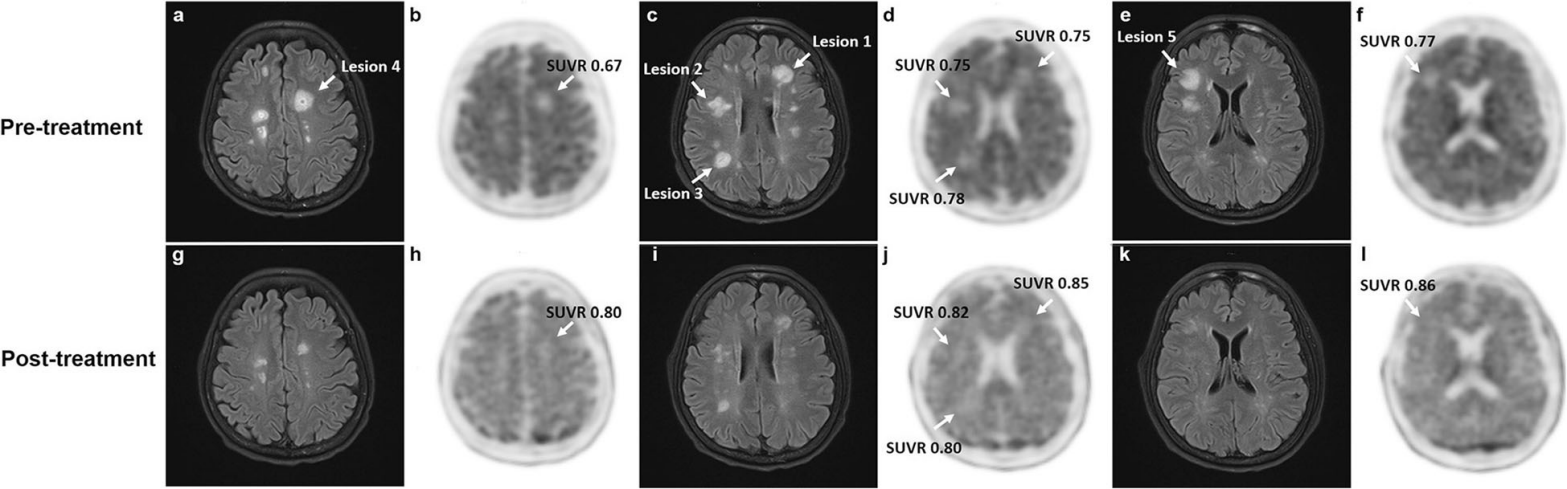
Demyelination and remyelination in five representative lesions on pre- and post-treatment ^18^F-florbetapir PET/MRI. a–f Pre-treatment PET/MRI showed multifocal hyperintense lesions (white arrow) with decreased ^18^F-florbetapir uptake (SUVR = 0.75, 0.75, 0.78, 0.67, and 0.77). g–l Five months later, reduced hyperintense lesions with increased ^18^F-florbetapir uptake (SUVR = 0.85, 0.82, 0.80, 0.80 and 0.86) were observed on post-treatment PET/MRI
